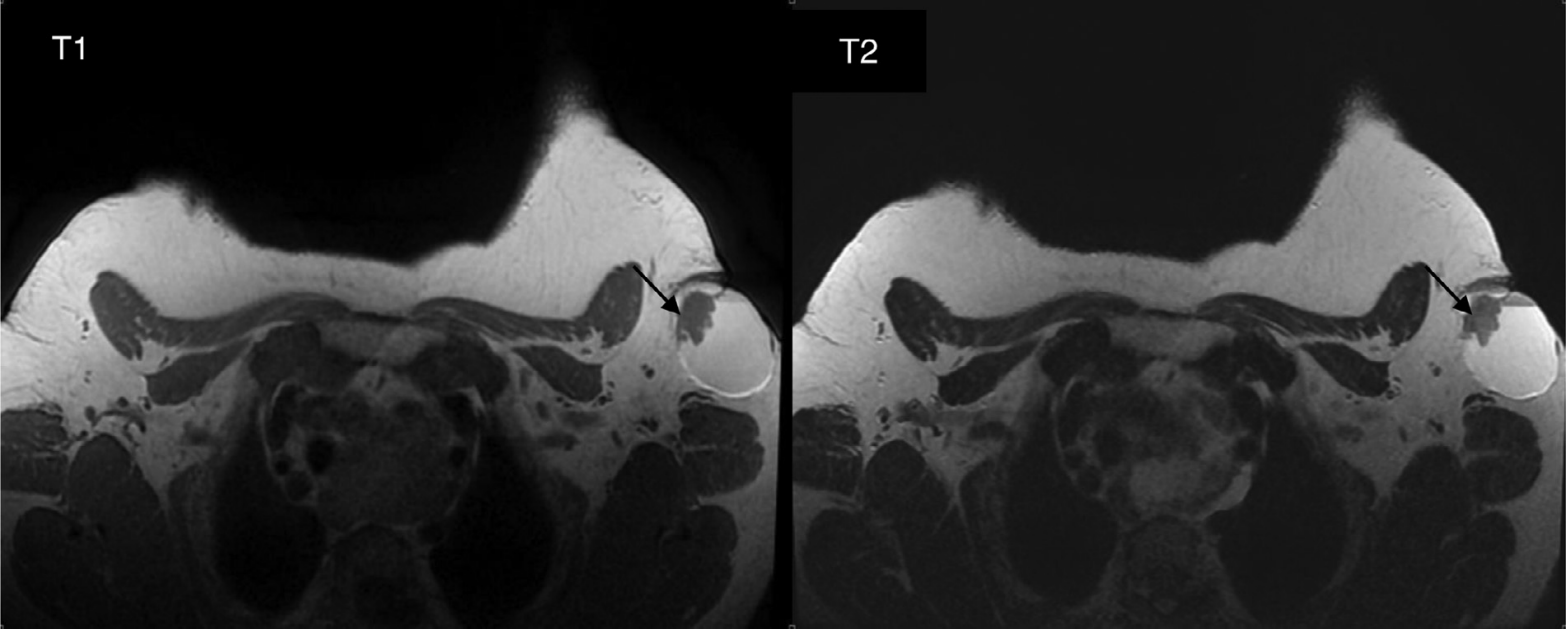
Axial T1- and T2-weighted images show the solid component (arrows) that appears hypointense in T1 with intermediate T2 signal.
